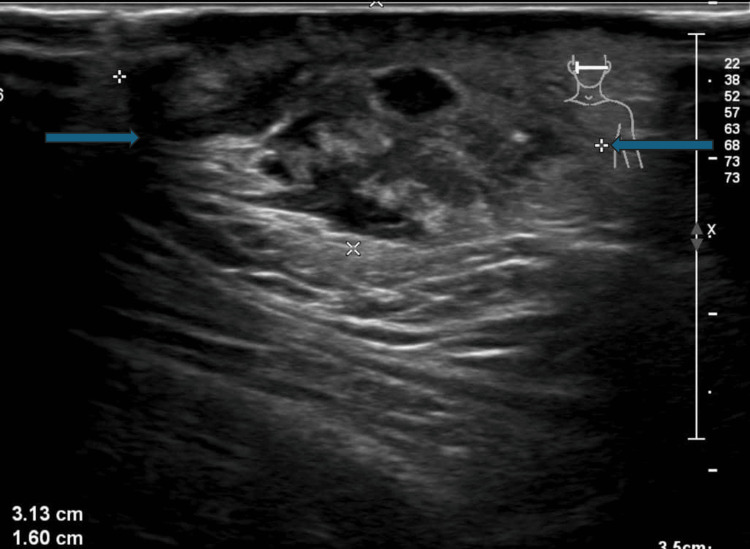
Follow-up ultrasonography of the lesion (June 2025). Ultrasound image showing a lesion with a significant interval increase in size, now measuring approximately 31 mm in maximum diameter (arrows). The lesion is located within the superficial subcutaneous tissue, in immediate contact with the overlying thickened skin and hypodermis. It appears as a heterogeneous, poorly defined complex area containing small anechoic fluid foci (largest approximately 4 mm) and hyperechoic components without posterior shadowing. Hypoechoic dermal and hypodermal tunnels are noted, suggesting dilatation of lymphatic ducts. Marked surrounding inflammatory oedema is evident.
